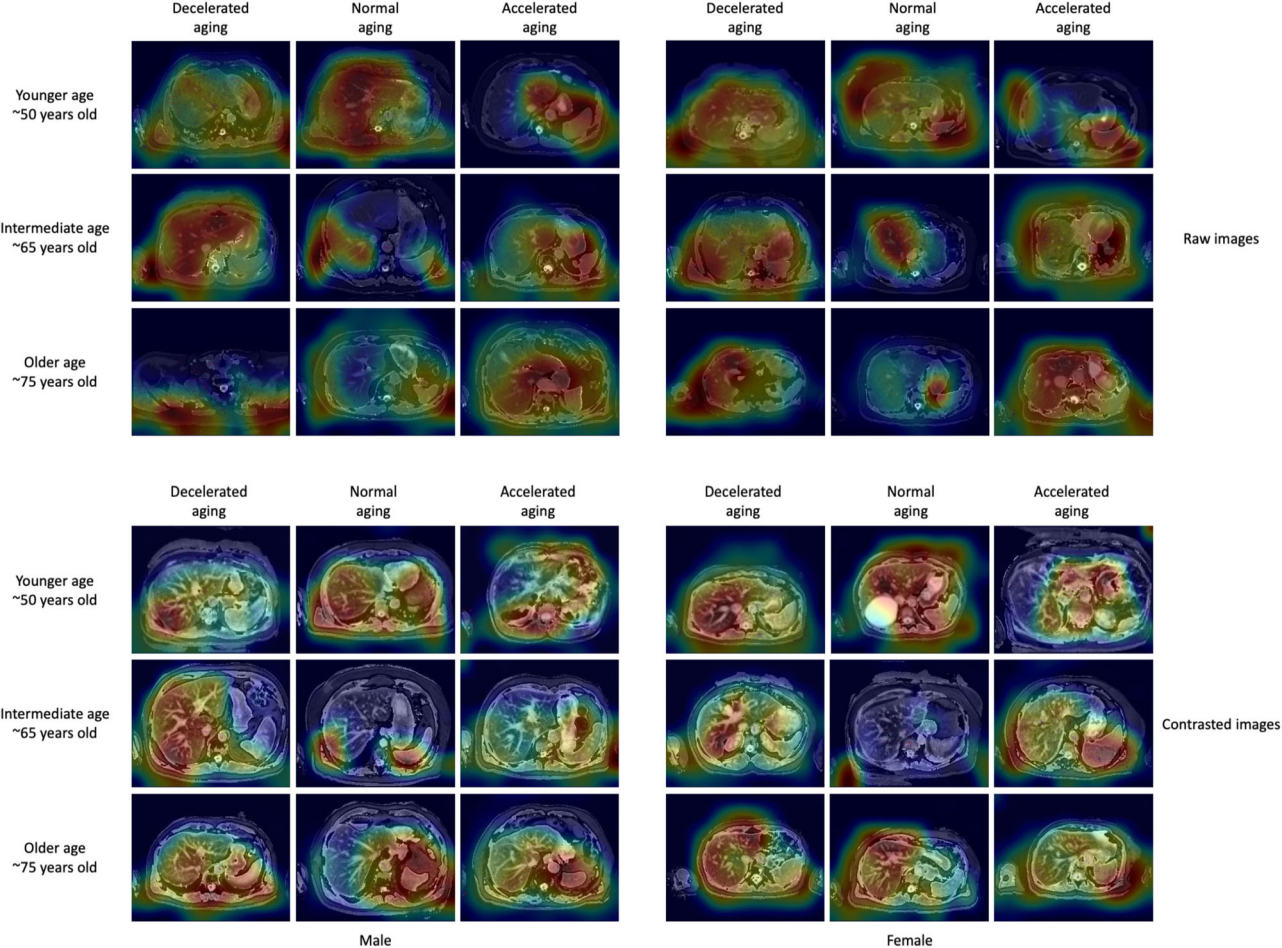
Sample attention maps for liver MRI-based models on raw and contrasted images. “Warm” filter colors (more red) highlight regions of high importance according to the Grad-RAM map. Actual chronological ages are obscured.
